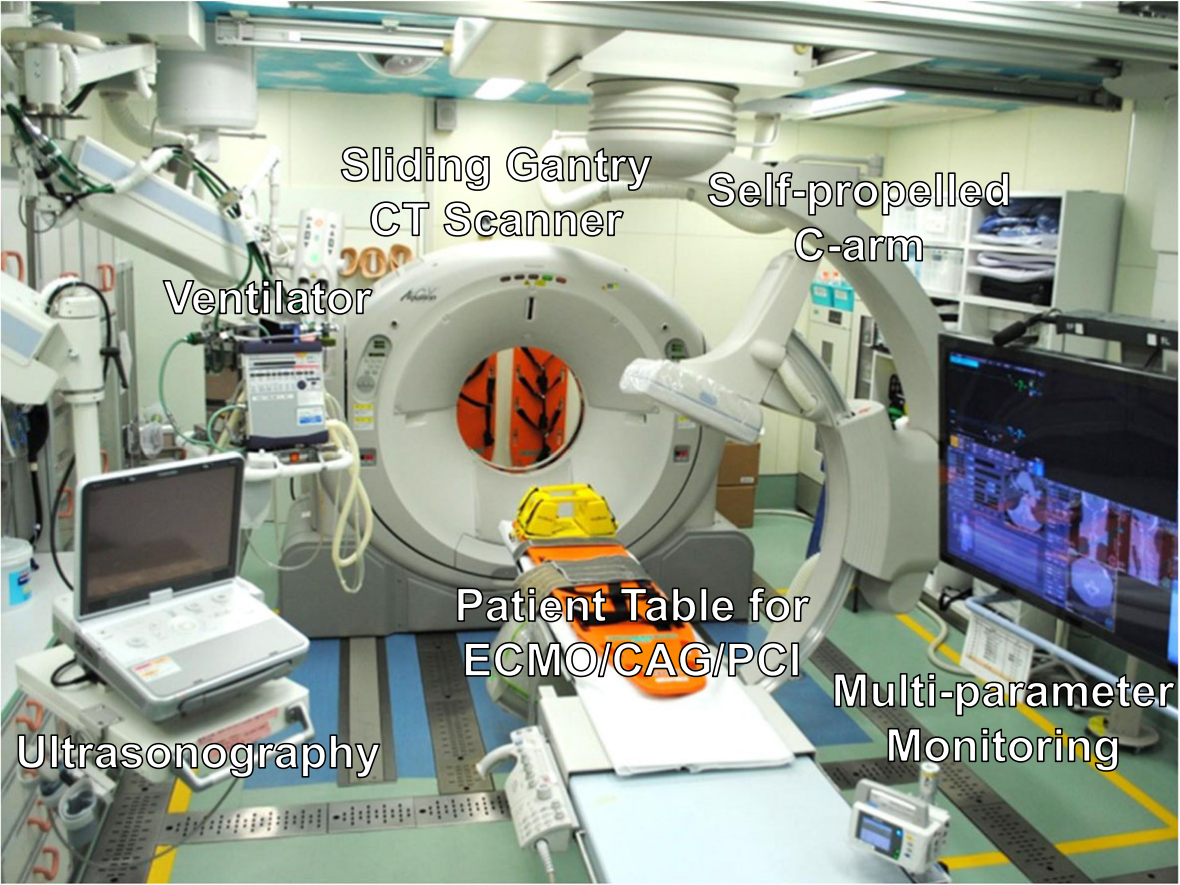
A photograph showing our IR-CT system in the emergency department. All life-saving procedures including airway management, emergency surgery, and transcatheter arterial embolization can be performed on the table without relocating the patient
